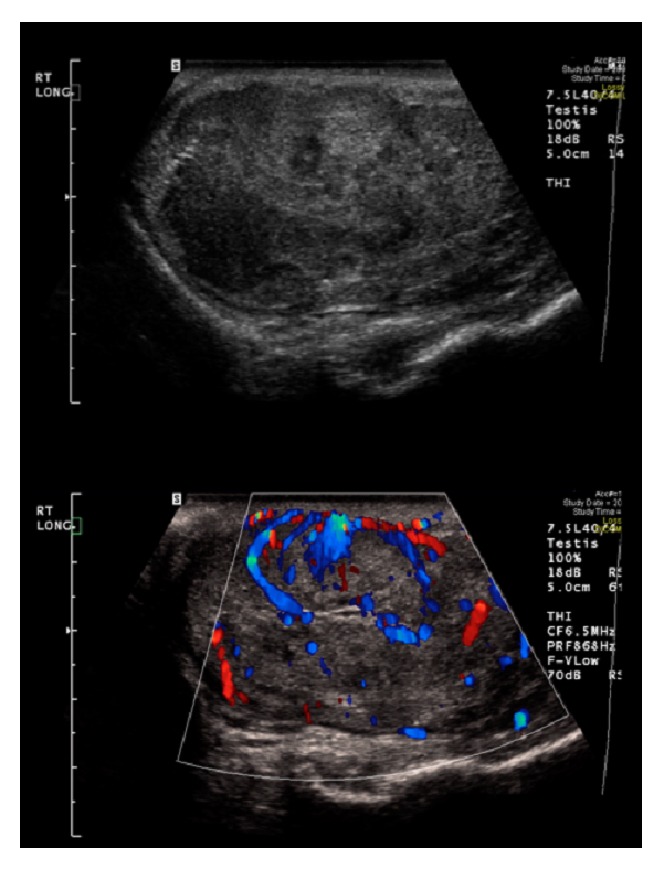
Right testicular ultrasound, showing a testicular mass with flow on Doppler.
